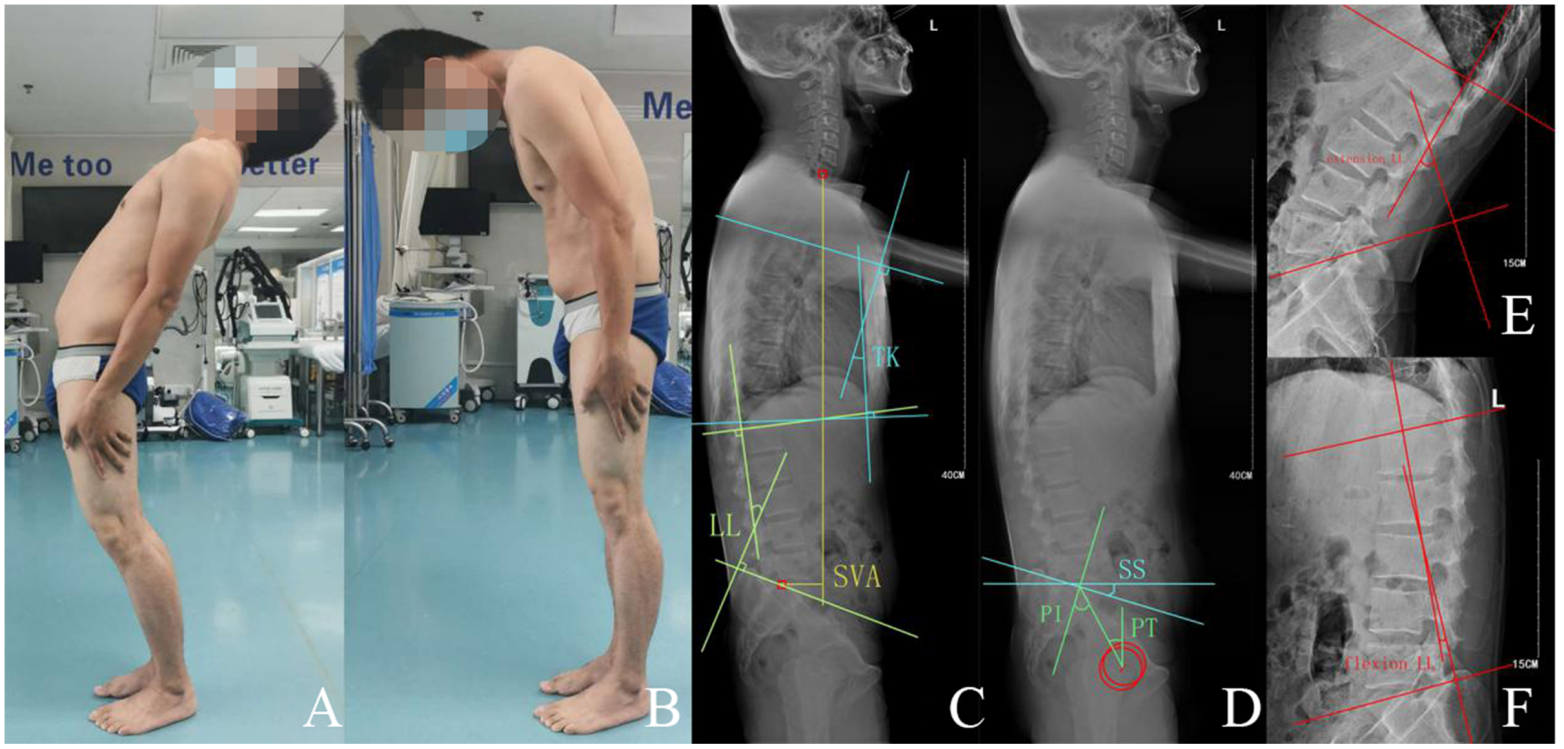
32 years old male with gluteal muscle contracture complained of low back pain and limitation of lumbar flexion. (A, B) Extension and flexion of lumbar, (C, D) standing lateral radiograph of spine-pelvis, SVA 61.6 mm, LL 15.4°, TK 12.8°, PT 21.6°, PI 41.3°, SS 19.7°, (E, F) extension-LL −50.2°, flexion-LL 5.4°.
